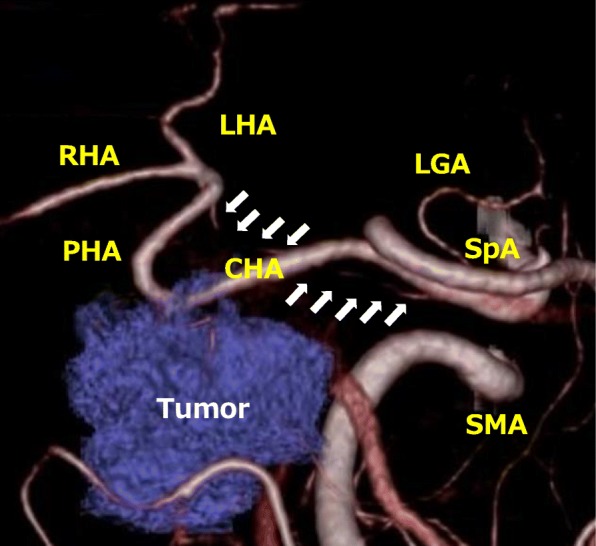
Three-dimensional CT angiography showed anastomosis between the root of the CHA and PHA (white arrow). RHA, right hepatic artery; LHA, left hepatic artery; LGA, left gastric artery; SpA, splenic artery; SMA, superior mesenteric artery
